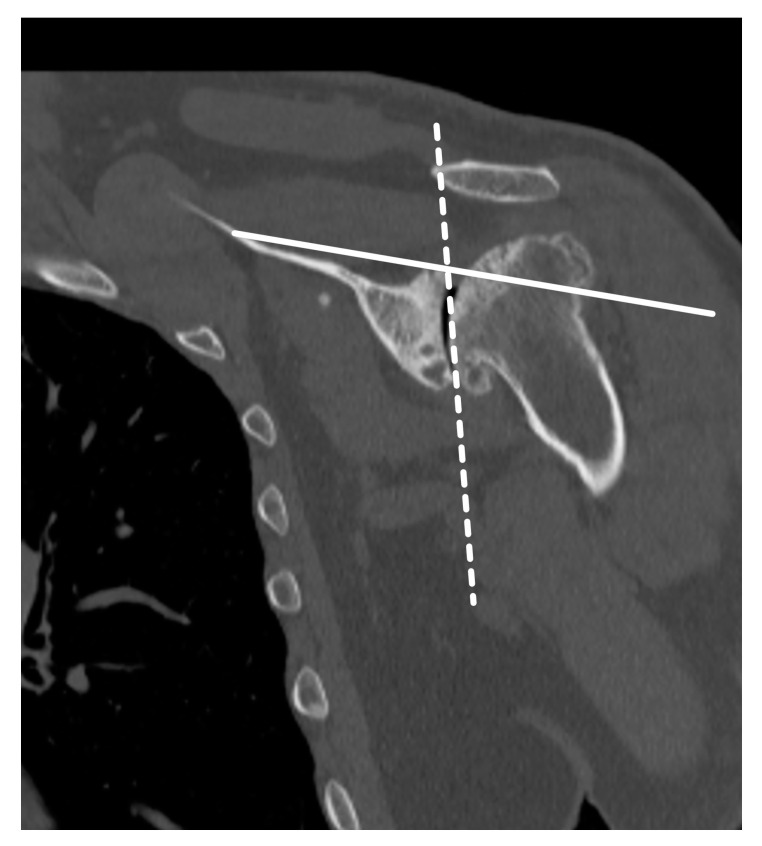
Coronal CT image showing glenoid inclination measurement. The inclination angle is measured between a line drawn along the supraspinatus fossa (solid line) and a second line along the glenoid fossa (dotted line).
